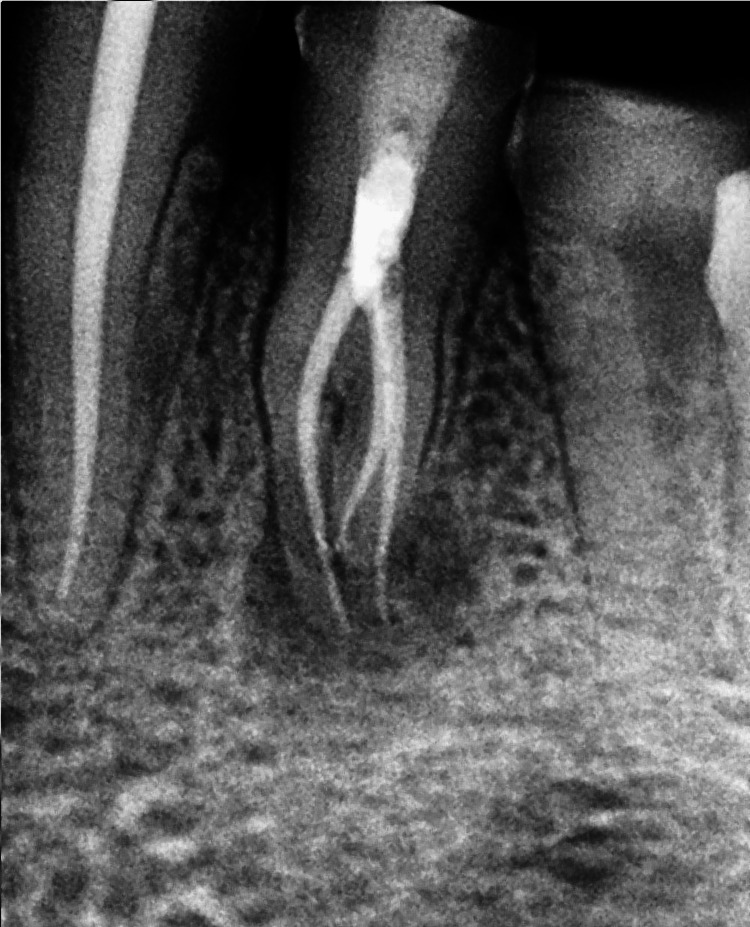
Post-obturation radiograph of mandibular left first premolar case 2
